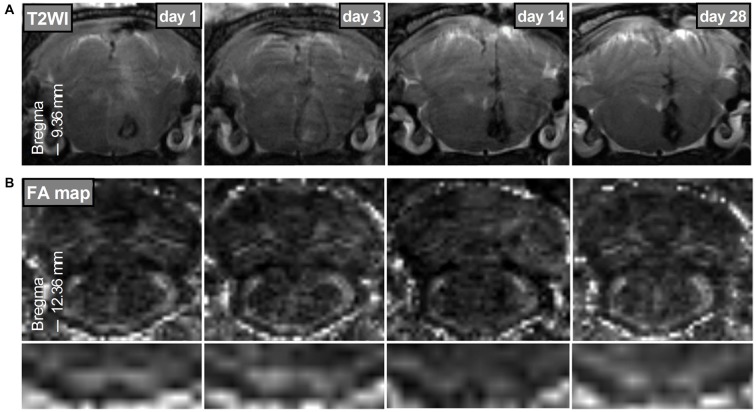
Bilateral PYs degeneration after brain stem hemorrhage (BSH). (A) T2-weighted images of the hematoma on days 1, 3, 14, and 28 after BSH. (B) FA maps of the bilateral PYs caudal to the hematoma (–12.36 mm of bregma) on days 1, 3, 14, and 28 after BSH. The bottom images are the enlarged FA maps of the bilateral PYs.
